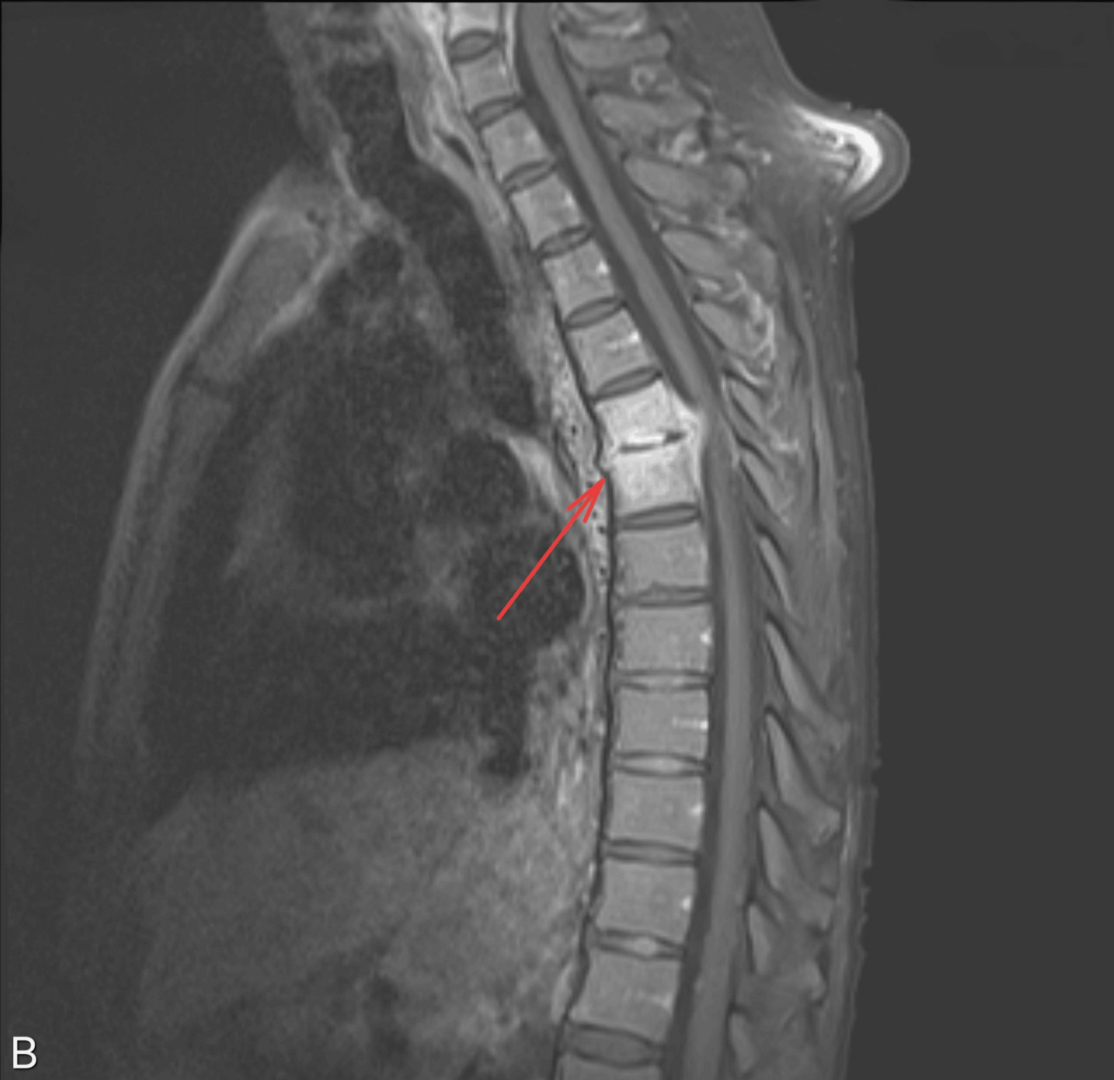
MRI of the thoracic spine with the red arrow showing osteomyelitis and evolving abscess at the level T5-T6
